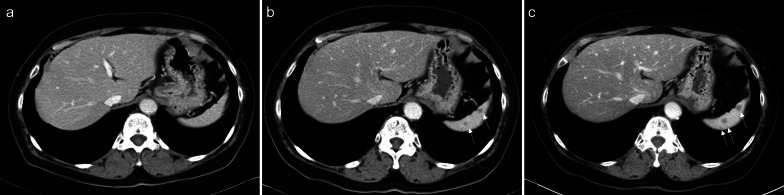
Time series findings in the spleen of case 1 obtained using CT. a No obvious splenic tumor is observed before the initial surgery. b CT images show several splenic tumors 3 years after the initial surgery. c Four years after the initial surgery, the splenic tumors are increased. *CT* computed tomography
